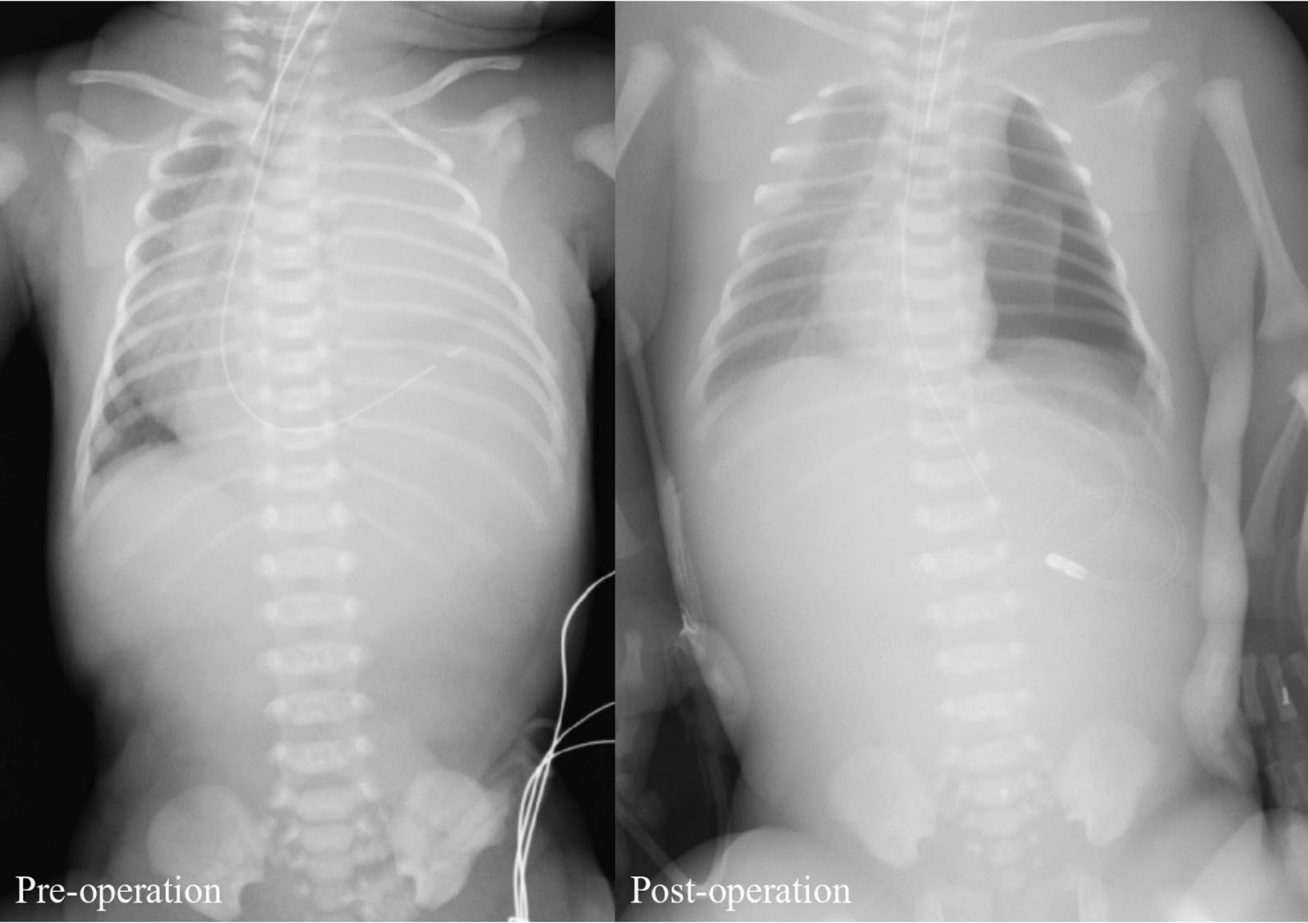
Chest radiograph showing a left diaphragmatic hernia. Preoperative chest radiograph reveals left diaphragmatic hernia. Postoperative chest radiograph reveals that the prolapsed organs are reduced
